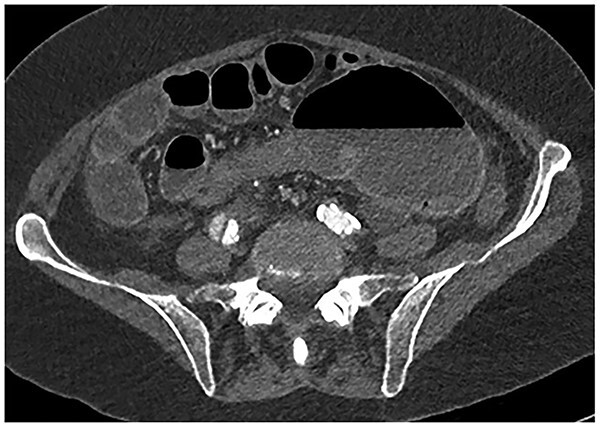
CT abdomen axial view demonstrating the Beaking sign—a progressive tapering of afferent and efferent limbs leading into the twist.
